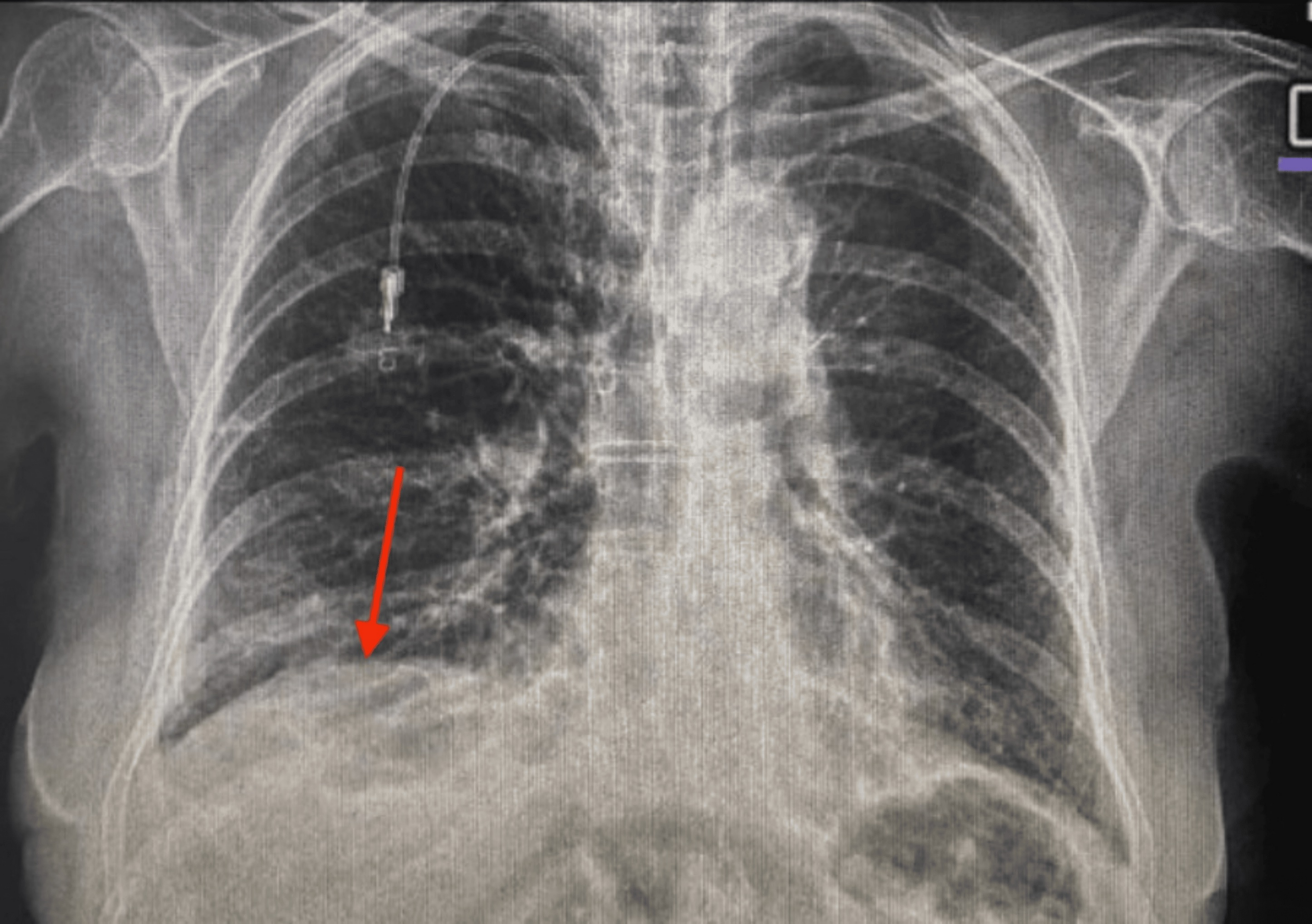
Chest X-ray following thoracentesis demonstrating residual right-sided effusion (red arrow) with interval bilateral improvement
